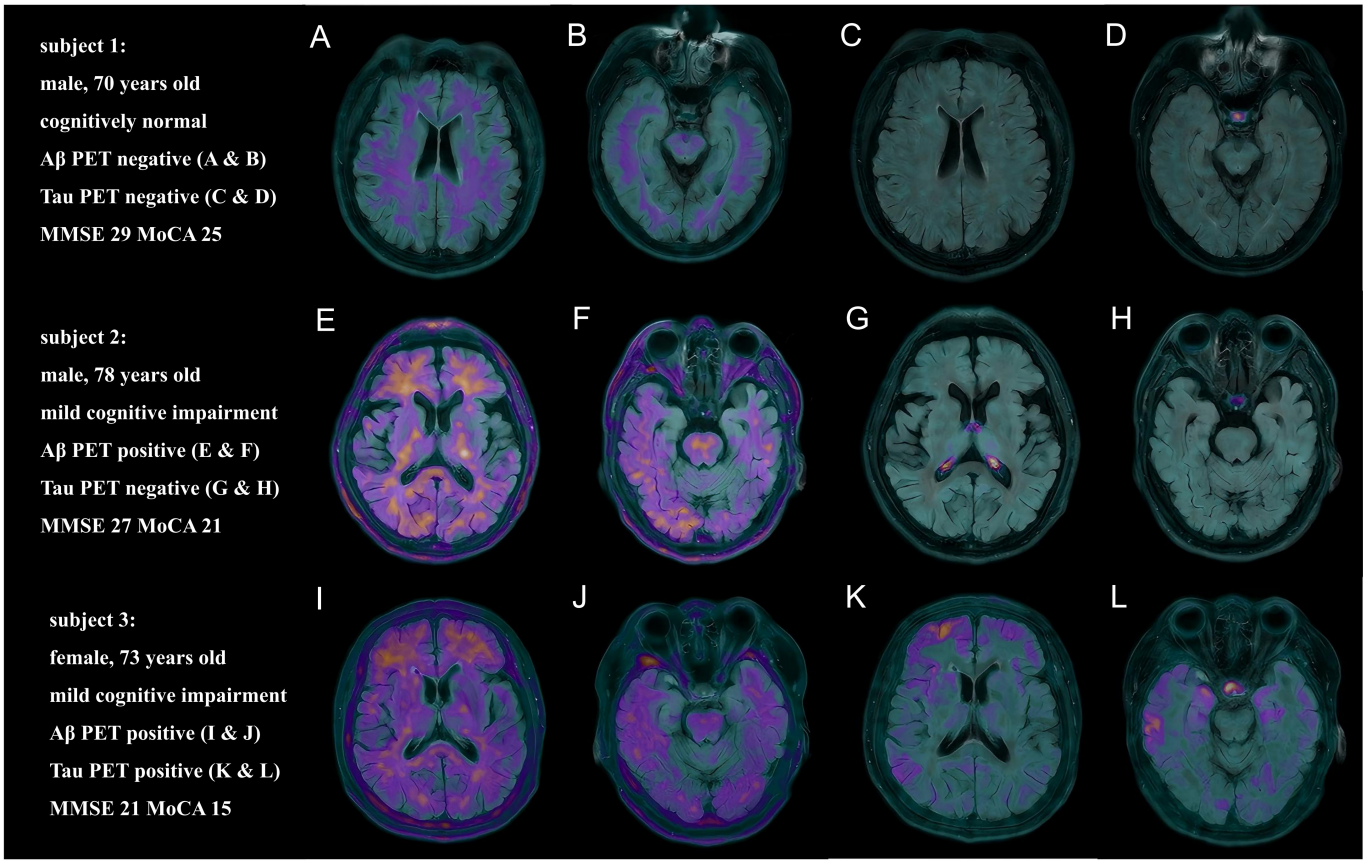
Representative ^18^F-AV45 PET and ^18^F-APN1607 PET uptake modalities for three participants in the PET cohort are shown. Subject 1: a cognitively normal male participant with no Aβ deposition on PET (A, B) and no tau deposition on PET (C, D); Subject 2: a male patient with mild cognitive impairment (MCI), with significant Aβ deposition (SUVR mean = 2.22) on PET (E, F) but no tau deposition (G, H), indicating stage A in the biological staging scheme for tau PET; Subject 3: an MCI female patient with significant Aβ deposition (SUVR mean = 2.01) on PET (I, J); moderate tau deposition (SUVR max = 1.88) was observed in both the medial temporal lobe and neocortical regions on PET, indicating stage C in the biological staging scheme for tau PET (K, L). Each panel reports age, sex, education, global cognitive status (cognitively normal or MCI), and scores on the Mini-Mental State Examination (MMSE) and the Montreal Cognitive Assessment (MoCA). Aβ, amyloid beta; PET, positron emission tomography; SUVR, standard uptake value ratio.
